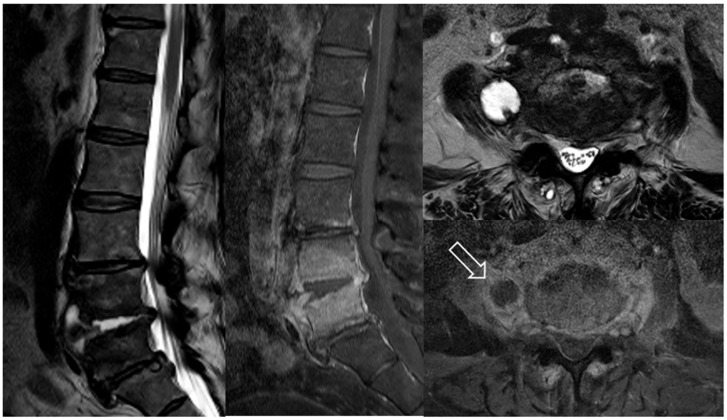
An 80-year old female patient presented to the emergency department with persistent lower back pain and fever. Magnetic resonance imaging(MRI) demonstrated signs consistent with spondylodiscitis at the L4–5 level, including disc space narrowing and endplate irregularity, as well as a rim-enhancing fluid collection in the right psoas muscle suggestive of an abscess. A white arrows indicate the location of the right psoas abscess on the axial image and the spondylodiscitis on the sagittal image.
